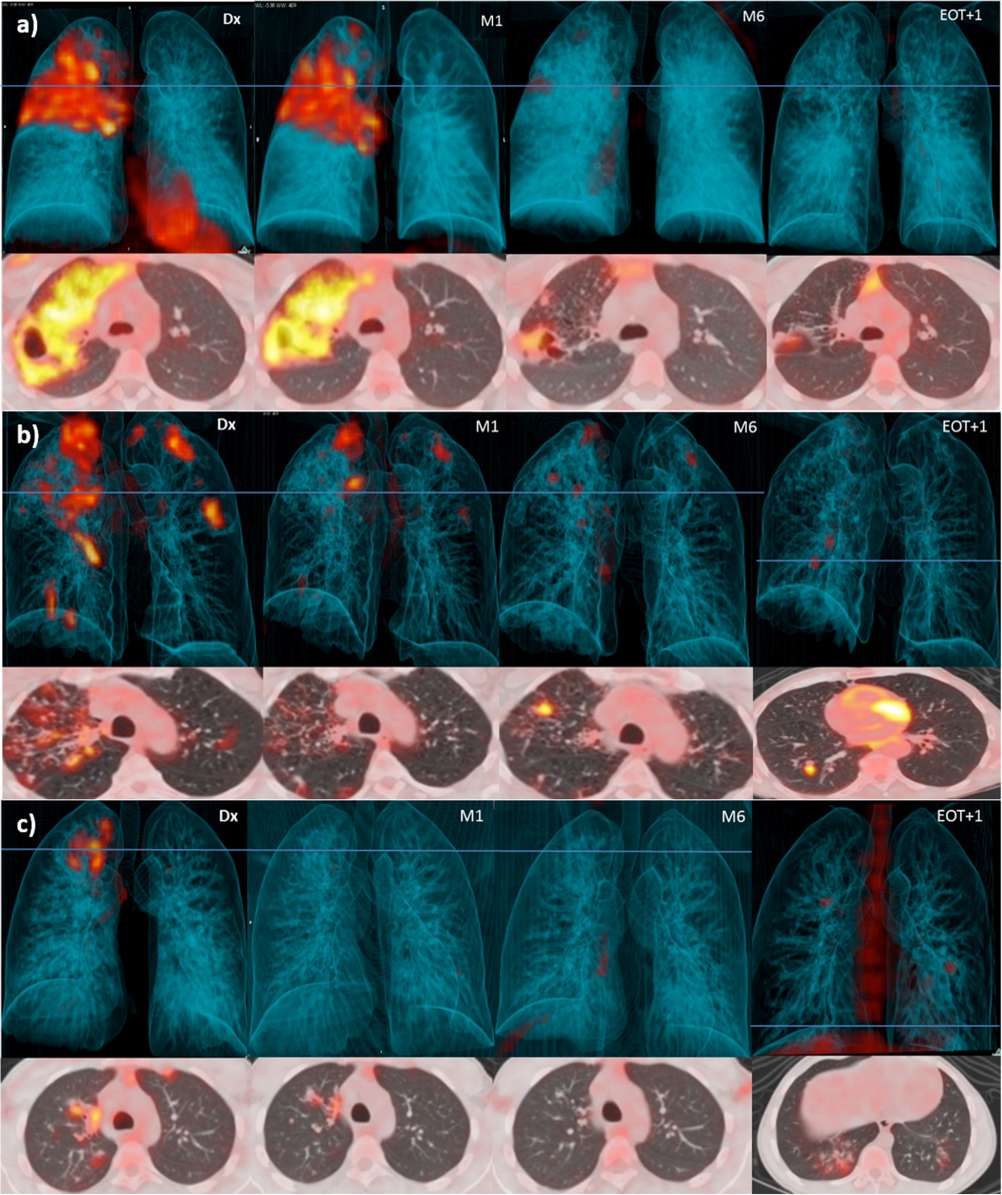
Dx, M1, M6 and EOT + 1y FDG PET-CTs for three representative cases that received 6 months of standard treatment and maintained cure. Three-dimensional anterior and transverse slices at the level of horizontal blue line. a Residual cavity with moderate FDG avidity at M6 improves over the next year, leaving nodular infiltrate with mild activity. b New nodule with high intensity seen at M6. It resolved at EOT + 1y, but two new nodules have formed. c All lesions resolved at M6, but three new areas with small nodular and tree-in-bud infiltrates seen at EOT + 1y
